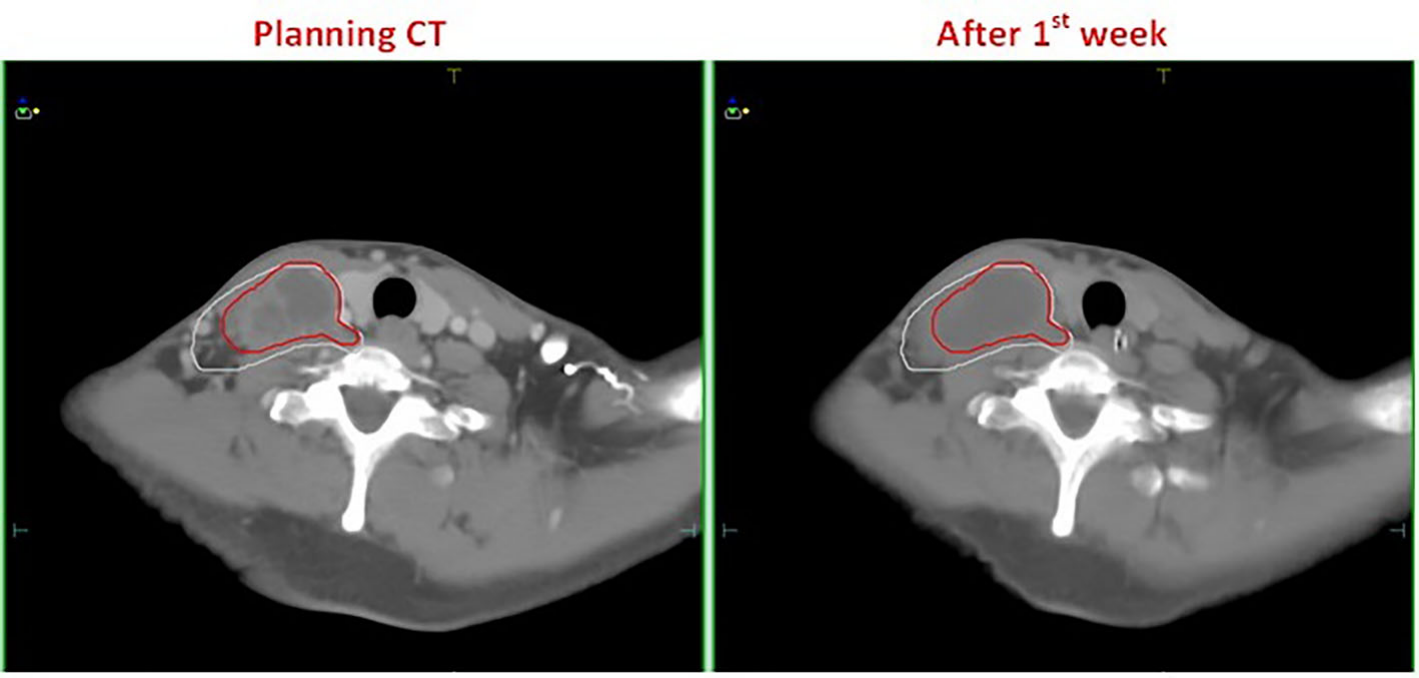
A patient with esophageal cancer who developed edema after the first week of radiotherapy. The left and right figures are the planning CT image and the repeat CT image after the first week of radiotherapy, respectively. The red line is the GTV contour at the planning stage, and the white line is the GTV contour after the first week of radiotherapy.
